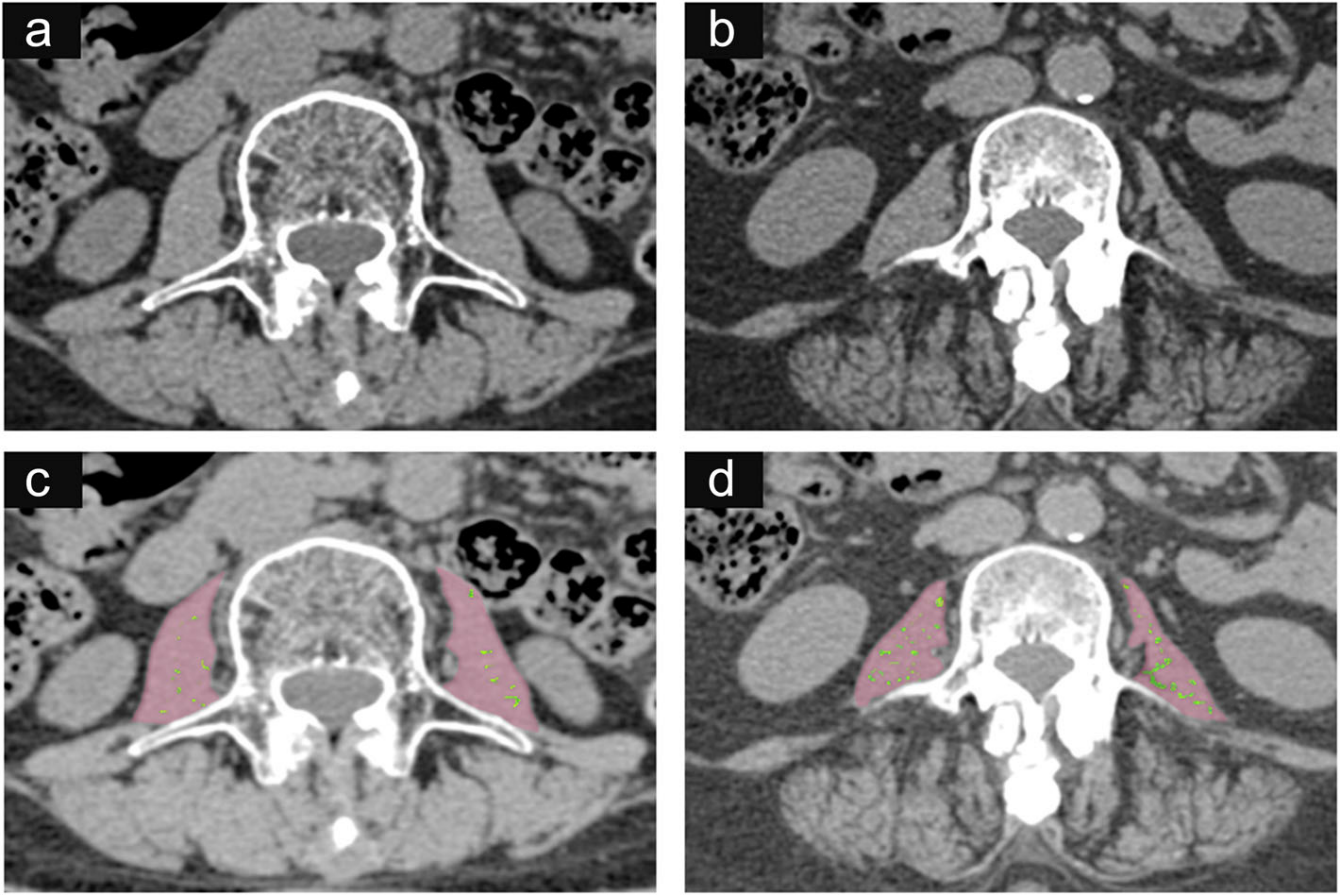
Measurement of the psoas. Images (a and c) are from a non-incisional hernia patient (64-year-old, female) and display a higher CT attenuation (47 HU) and a lower fatty infiltration rate (green, 2.1%); images (b and d) are from an incisional hernia patient (67-year-old, female) and exhibit a lower CT attenuation (24 HU) and a higher fatty infiltration rate (green, 9.4%). Their psoas muscle indexes are roughly equal (4.0 cm^2^/m^2^ vs. 4.1 cm^2^/m^2^)
